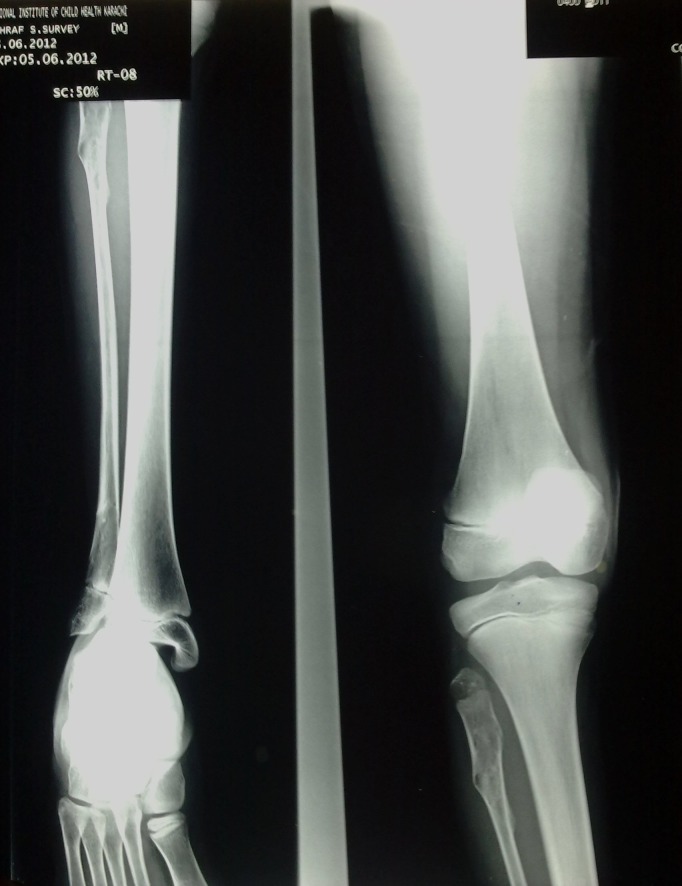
Figure 5: Plain radiograph – left lower limb: enchondromas involving proximal and distal fibula.
